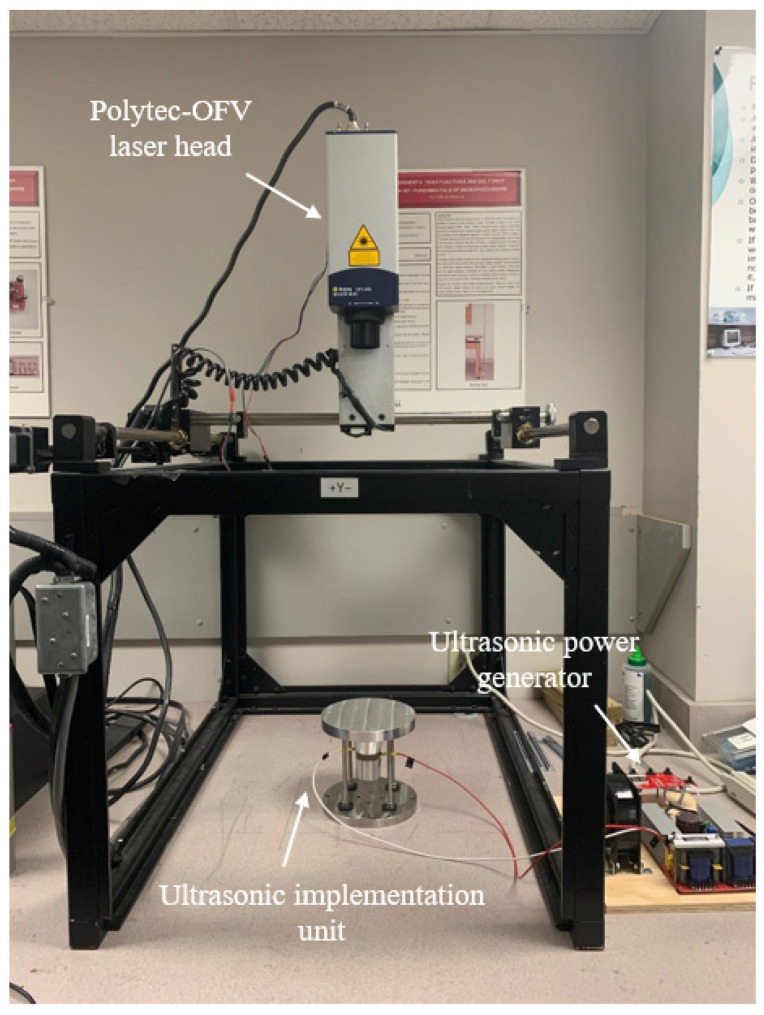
Experimental setup for ultrasonic velocity measurement.
